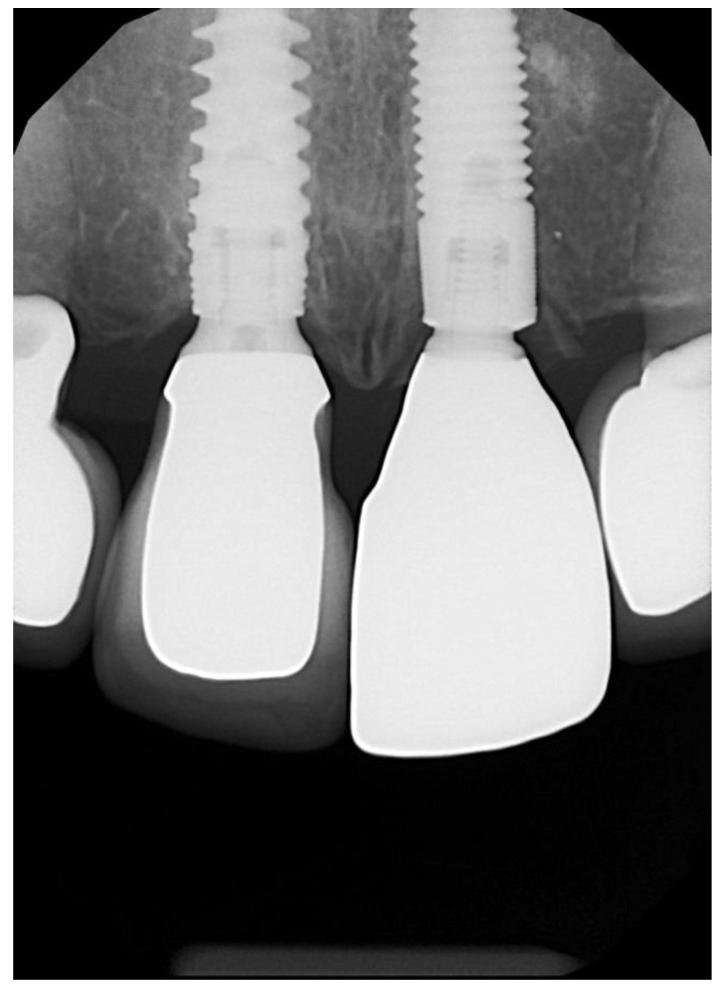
X-ray of final crown confirming correct seating and no interference with the shield.
